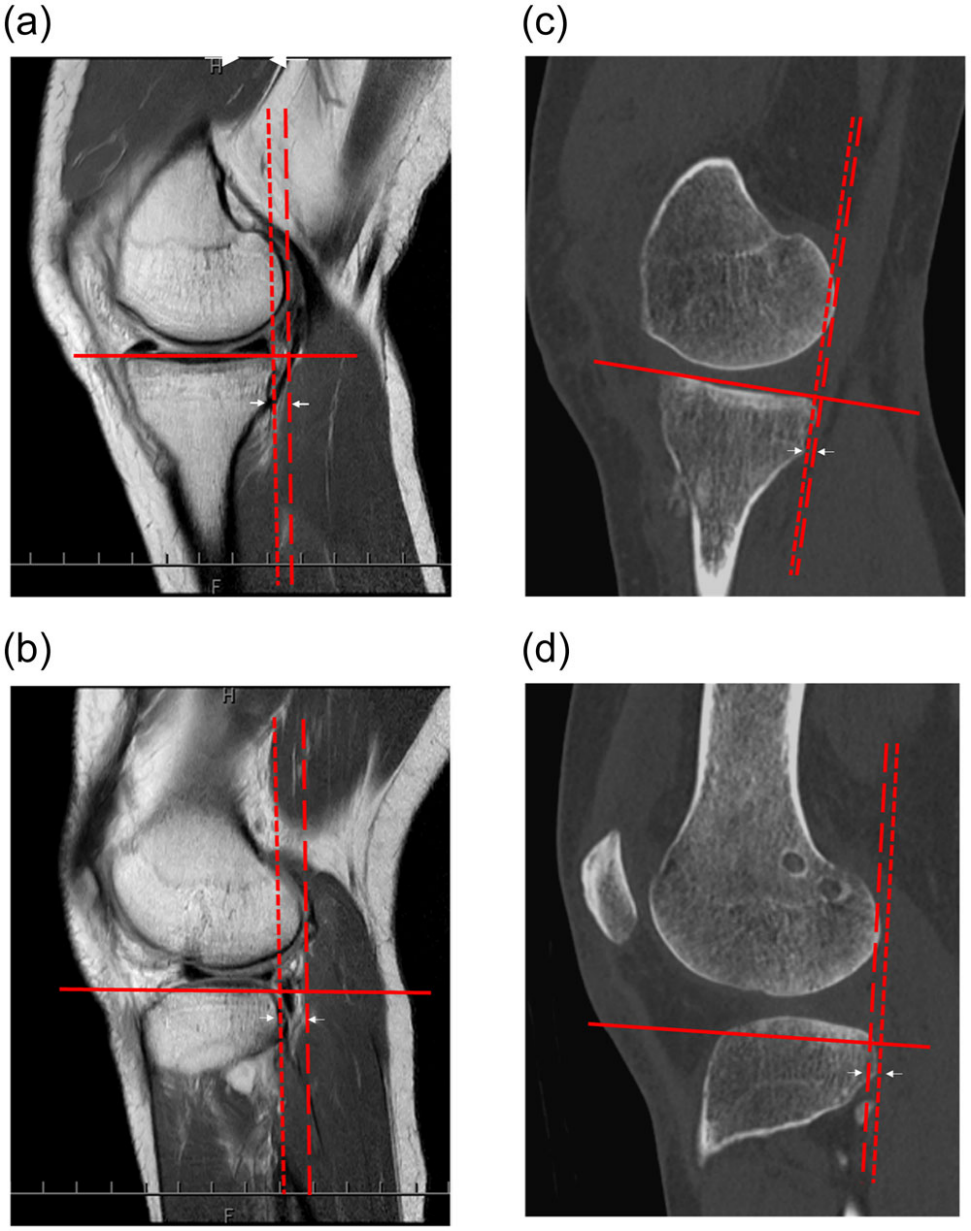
Measurement of the anterior tibial translation (ATT) on magnetic resonance imaging (MRI) and computed tomography (CT). The ATT is assessed using sagittal sequences. It is defined as the distance between the vertical line drawn to the posterior edge of the centre of each femoral condyle (dashed line) and the vertical line drawn to the posterior edges of each tibial plateau (dotted line). A positive value indicates the anterior translation of the tibia relative to the femur (arrow). (a) Measurement of medial ATT on MRI. (b) Measurement of lateral ATT on MRI. (c) Measurement of medial ATT on CT. (d) Measurement of lateral ATT on CT.
